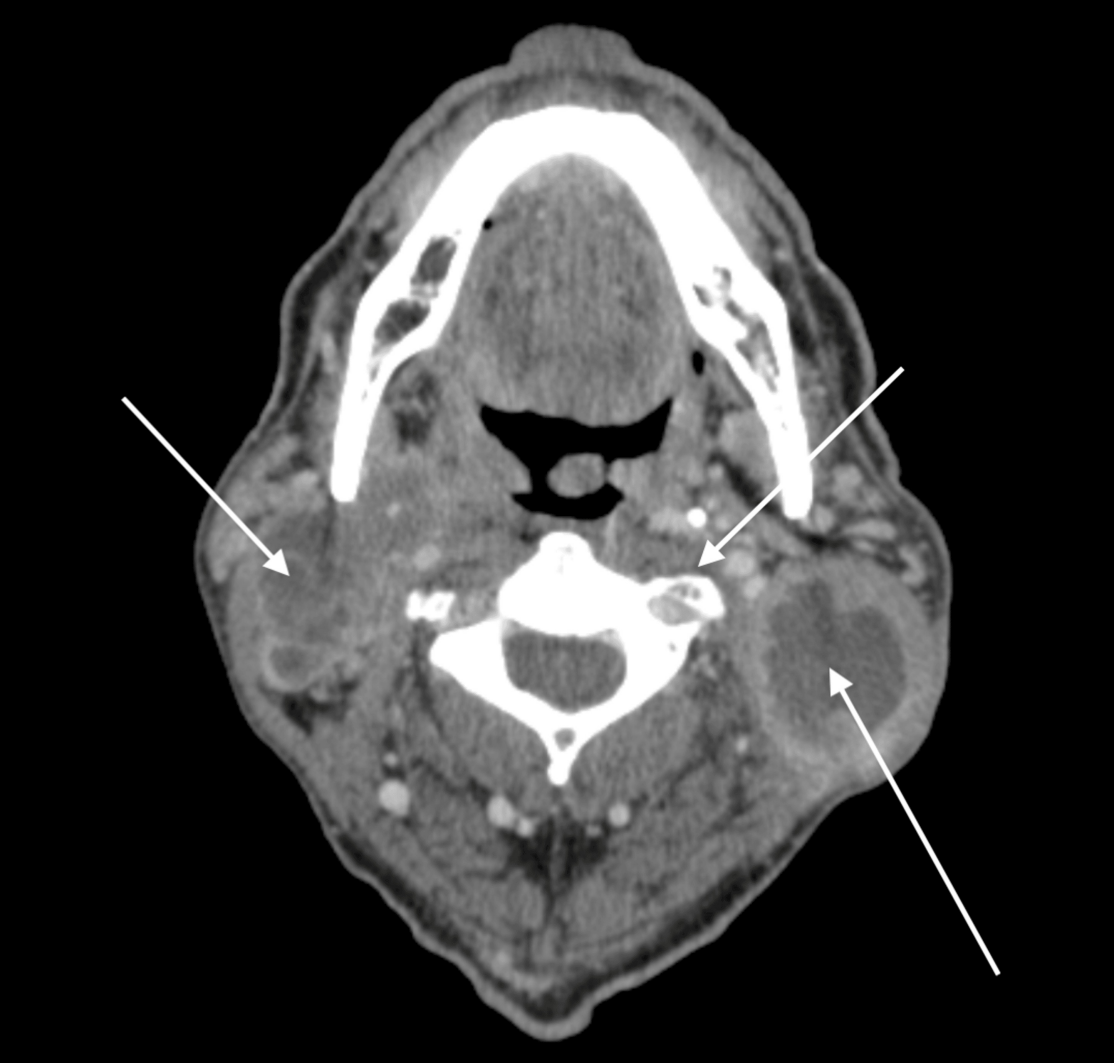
Axial view of head CT scan showing a hypodense laryngeal mass measuring 2.4 x 1.0 x 2.3 cm extending inferiorly into the left aryepiglottic fold and left vocal cord and extensive bilateral necrotic cervical lymphadenopathy measuring up to 5.7 x 3.6 cm
